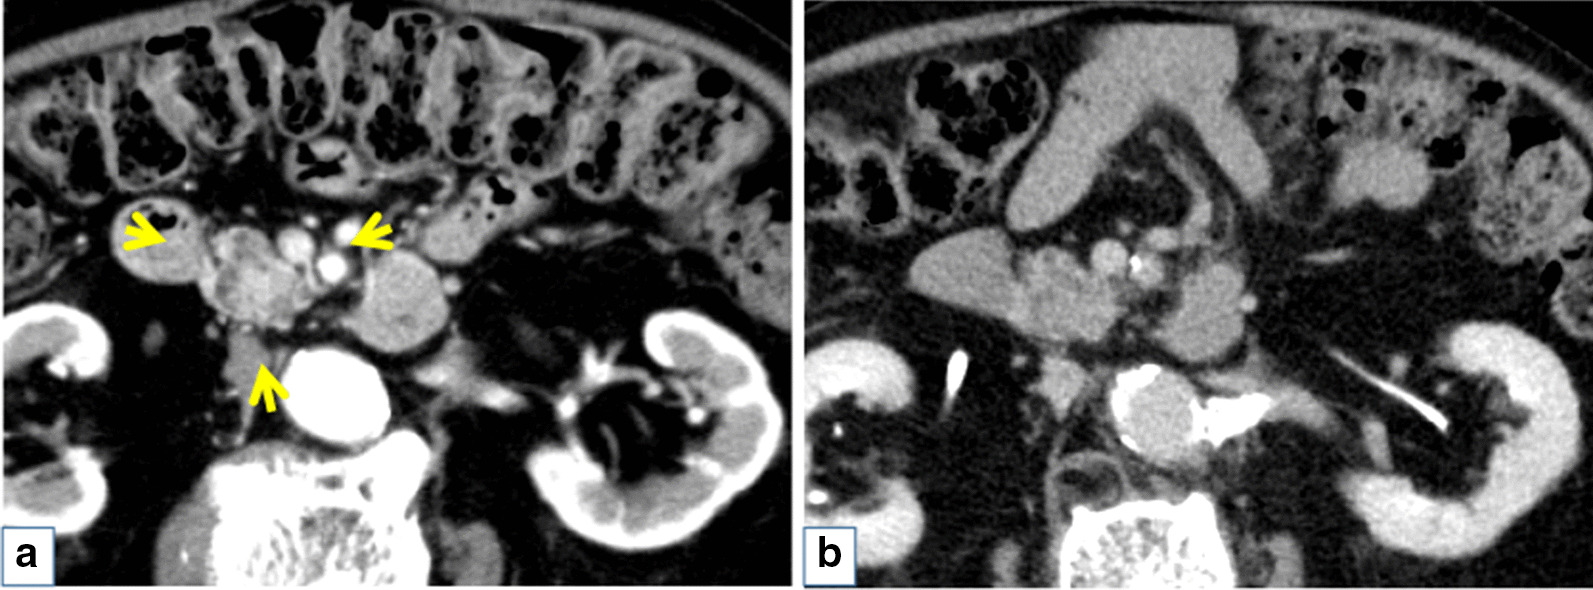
Contrast enhanced computed tomography revealed enhanced round mass sized in 15 mm surrounding with a little fluid (arrow) in early phase (a) and the mass was not clearly detected in equilibrium phase (b)
